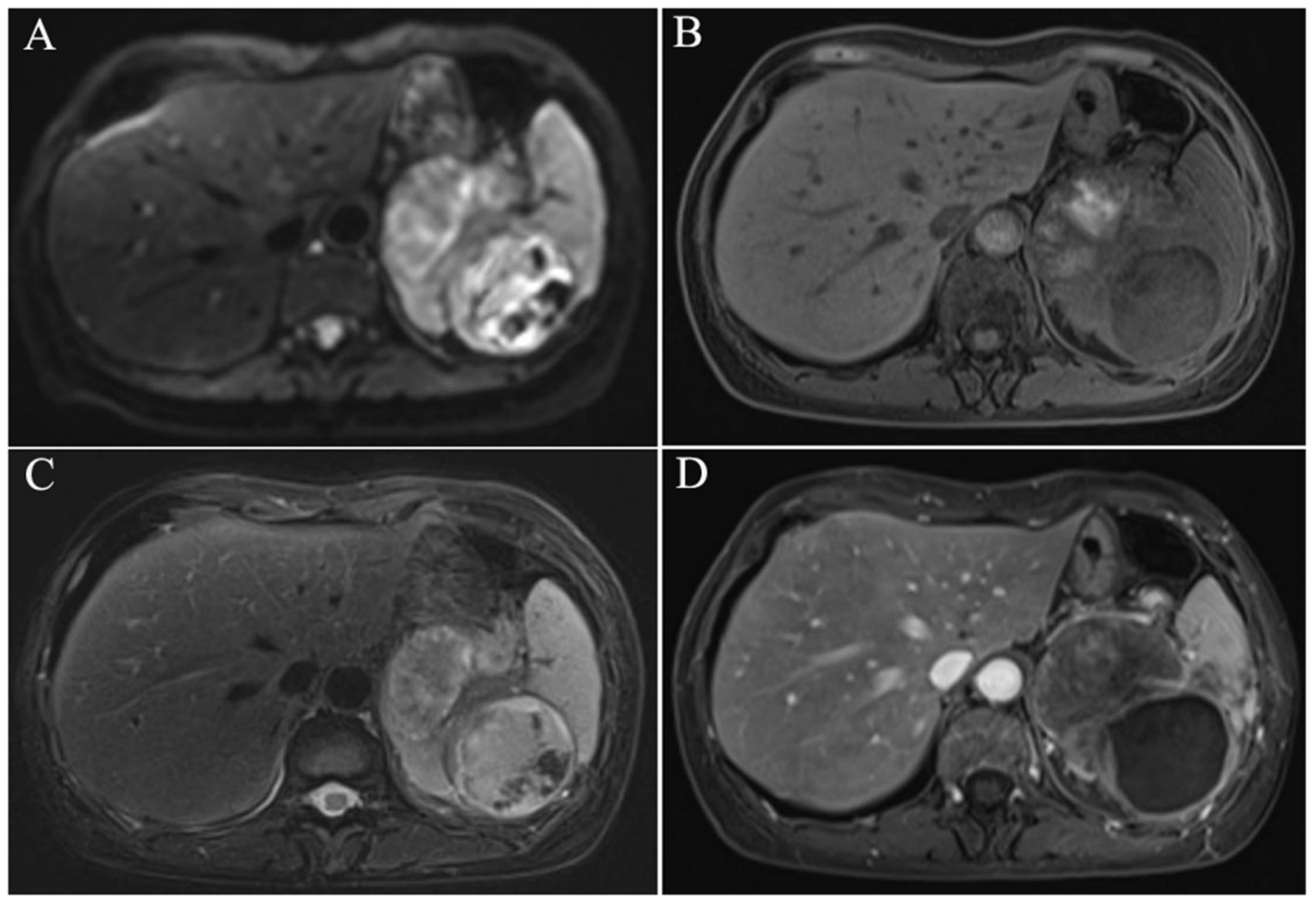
MRI revealed a mixed solid–cystic lesion with heterogeneous enhancement of the mural nodules and septations [(A) diffusion-weighted imaging, (B) T1-weighted imaging, (C) T2-weighted imaging, (D) Venous phase].
